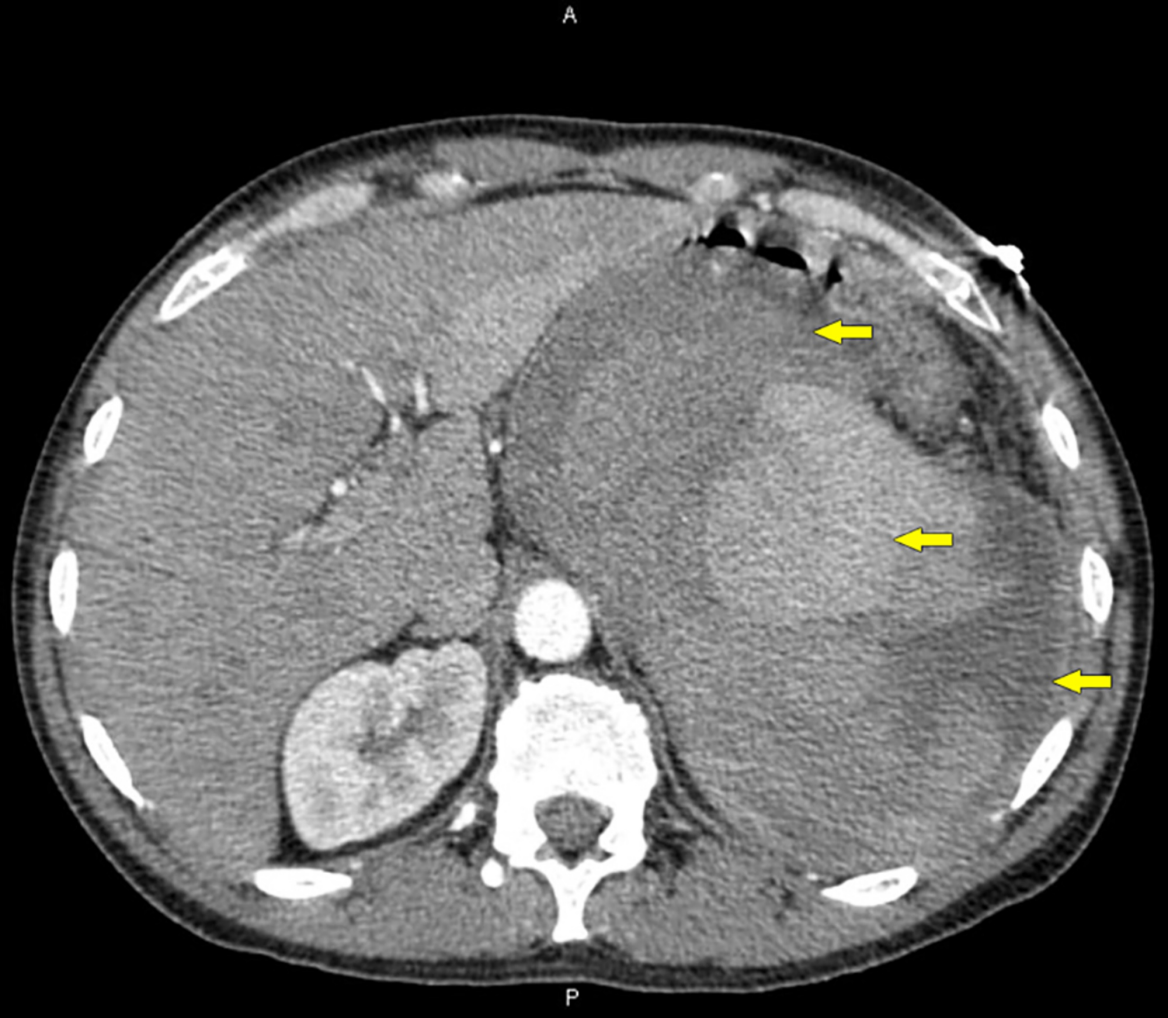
New CTA of abdomen showing huge variegated hematoma (yellow arrows) suggesting recent hemorrhage. CTA, CT with angiography
